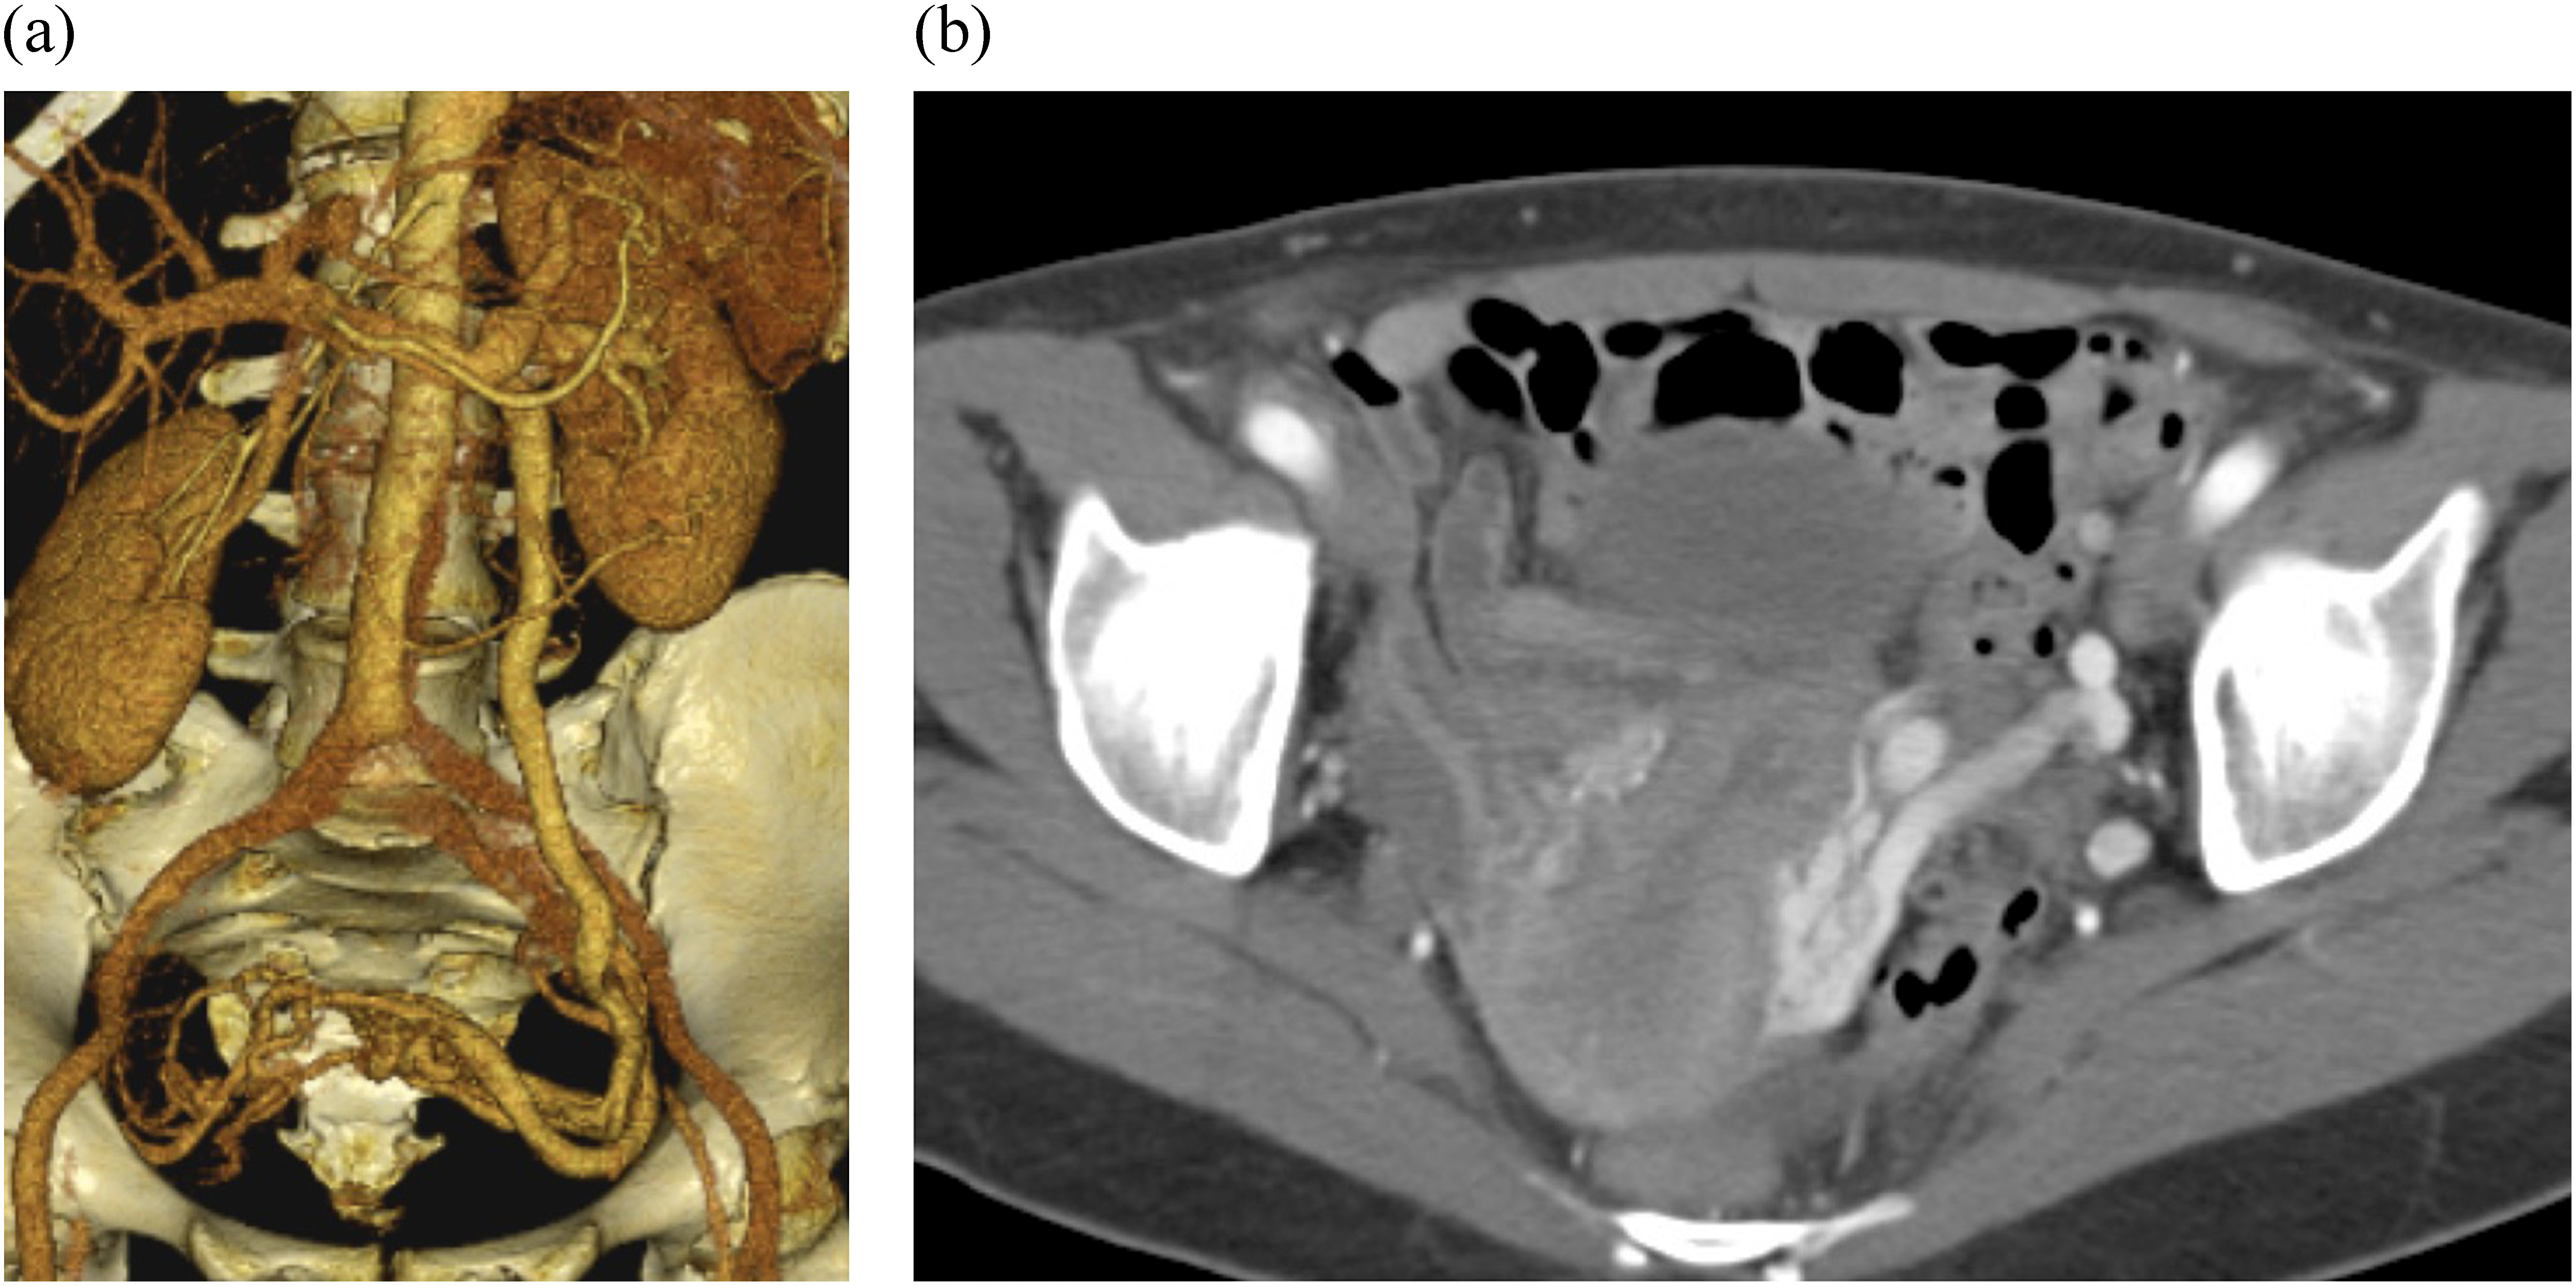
Fig. 1 Preoperative computed tomography scan images showing compression of the left renal vein with dilation of the left gonadal vein.
